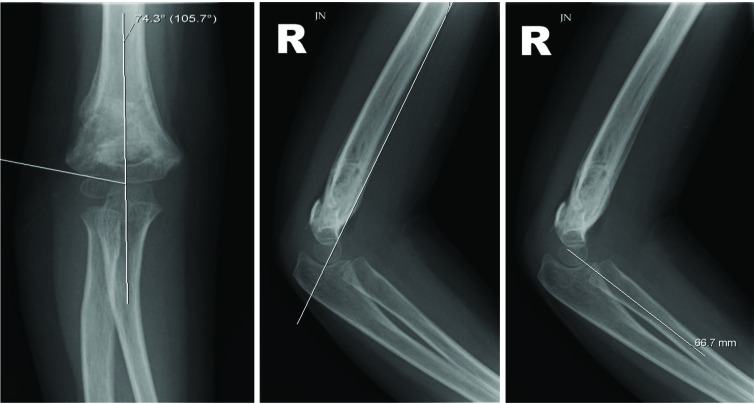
The radiographs show the calculation of Baumann’s angle (a), and the assessment of the anterior humeral line (b) and the radiocapitellar line (c).
